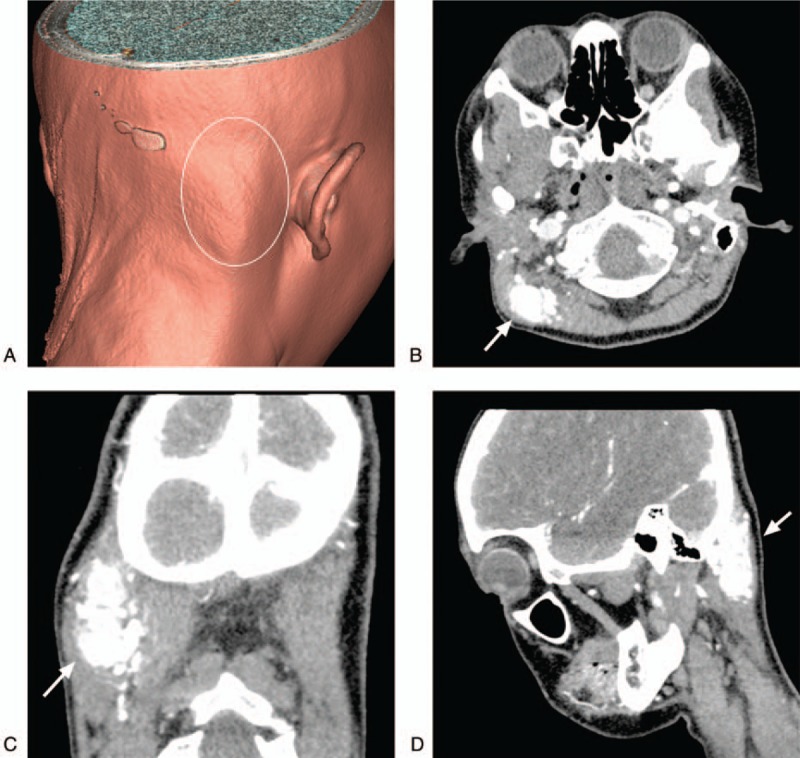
Preoperative CT and 3D reconstruction images. A: A 3D reconstructed CT showing the lesion in the right occipital region with a slight bulge in the skin (white circle). B–D: CT enhanced images of axial, coronal and sagittal planes showing that the lesions were mainly vascular in nature, presented as a “wool mass”, were located in a subcutaneous region, involved the muscles, and showed obvious enhancement (arrow).
